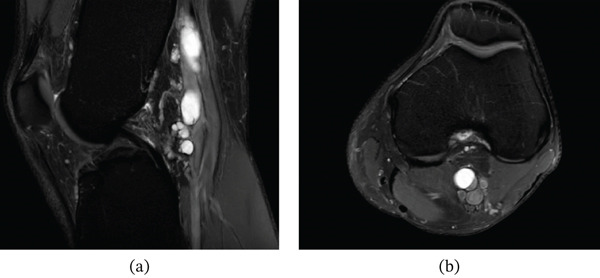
Sagittal (a) and axial (b) T2‐weighted MRI images of the knee revealing a multiloculated, homogeneous cystic lesion extending proximally adjacent to the popliteal artery, causing compression of the surrounding structures.
